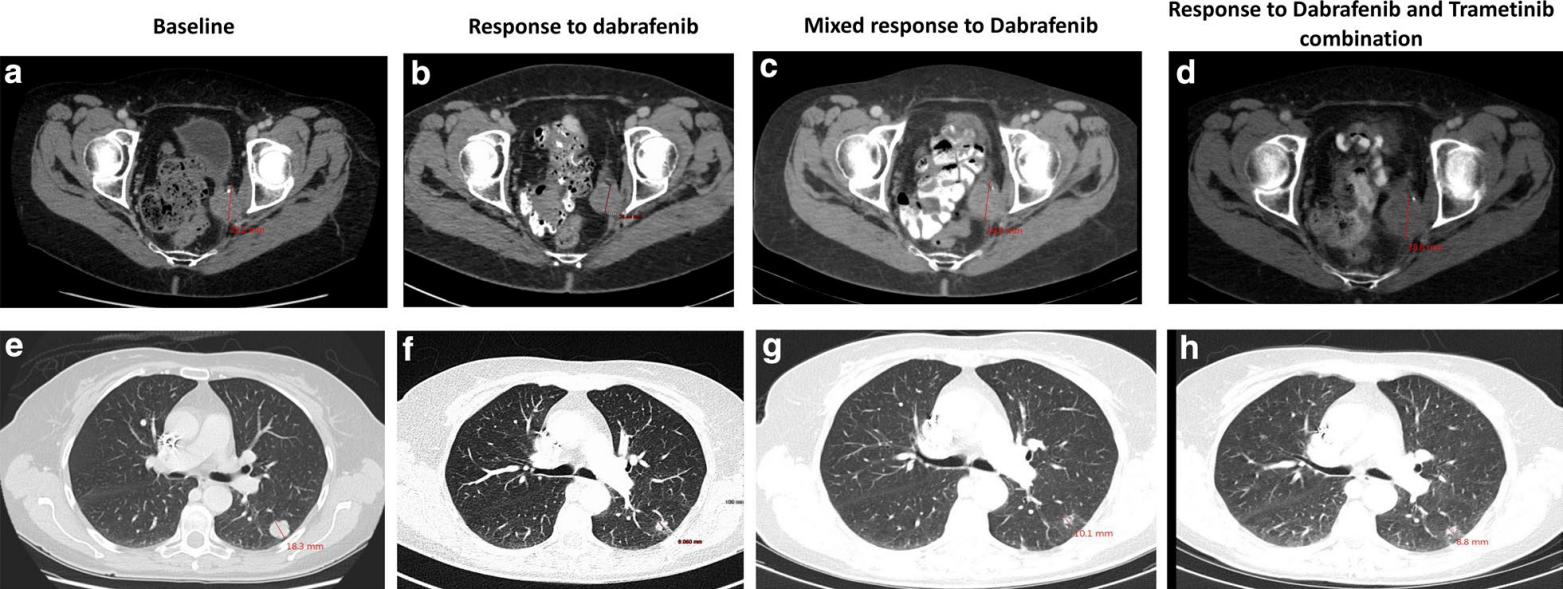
Representative CT scan images of target lesions showing response to dabrafenib monotherapy and dabrafenib and trametinib combination. Patient started treatment and showed response at the first 3 monthly CT scan (b, f) in all target lesions compared to the baseline (a, e). At the second 3 monthly CT scan the pelvic mass showed increased size (*panel*
